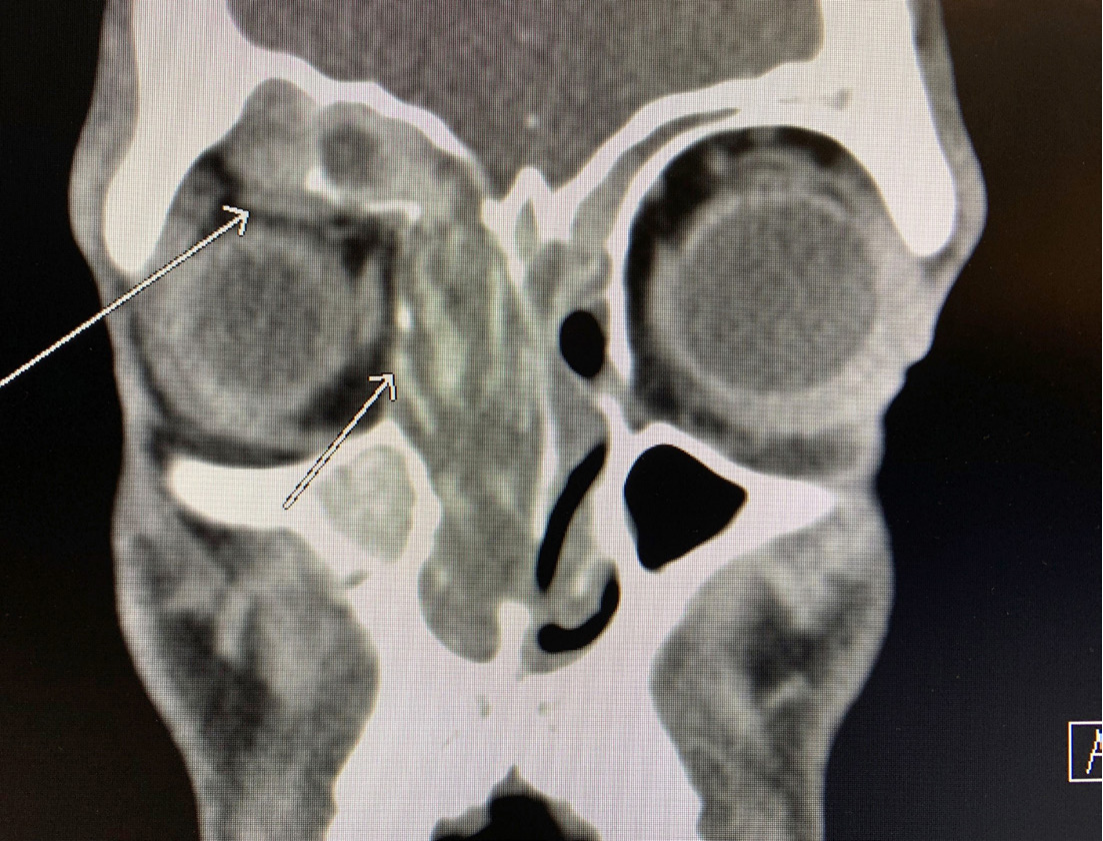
Computed tomography demonstrating allergic fungal sinusitis. The shorter arrow notes destruction of orbital wall, with longer arrow demonstrating compression of superior rectus muscle.
